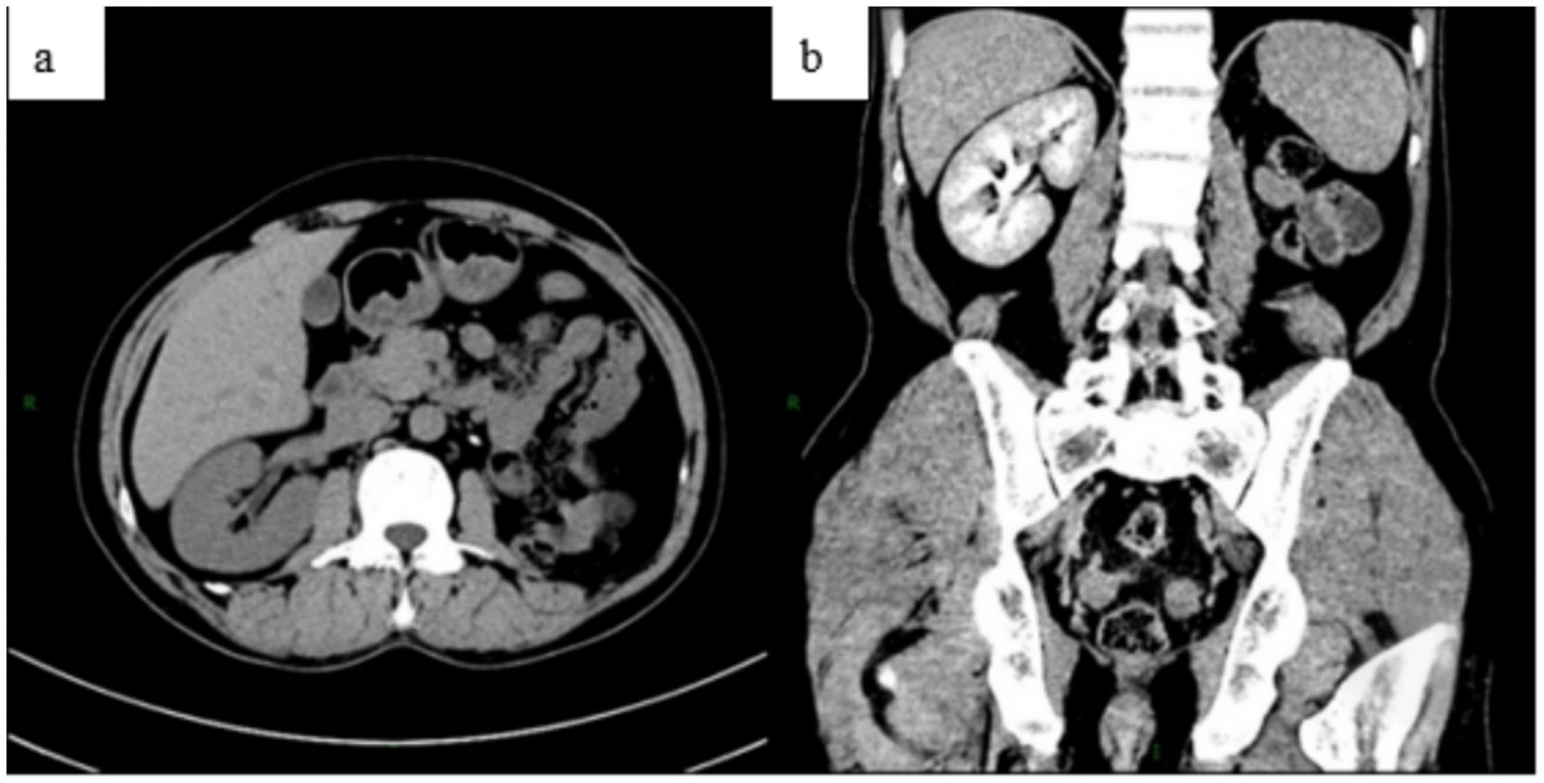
Abdominal CT was reviewed 3 months after surgery. The plain scan (a) and enhanced scan (b) showed no obvious abnormalities following the left nephrectomy.
